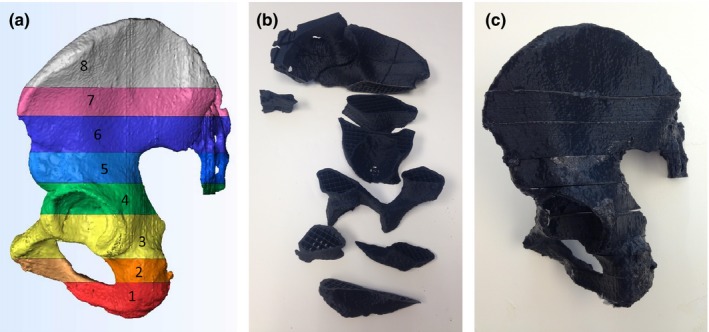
CT‐derived half‐pelvis model shown as (a) CAD‐based model cut into 8 components for 3D printing, (b) 3D printed components (partially assembled and flipped to show reverse side), and (c) fully assembled half‐pelvis model.
